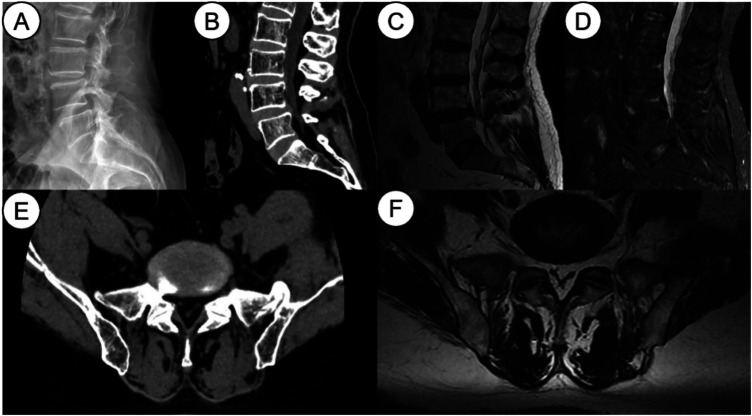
(A–D) Results of imaging examinations performed on the patient upon admission (lateral x-ray of the lumbar spine, lumbar spine CT, lumbar spine MRI T2, and T2 fat suppression sequences); (E) and (F) show the transverse CT and MRI T2 sequences of the L5-S1 segment, where nerve dura deformation due to fat compression can be observed, forming a “V”.
